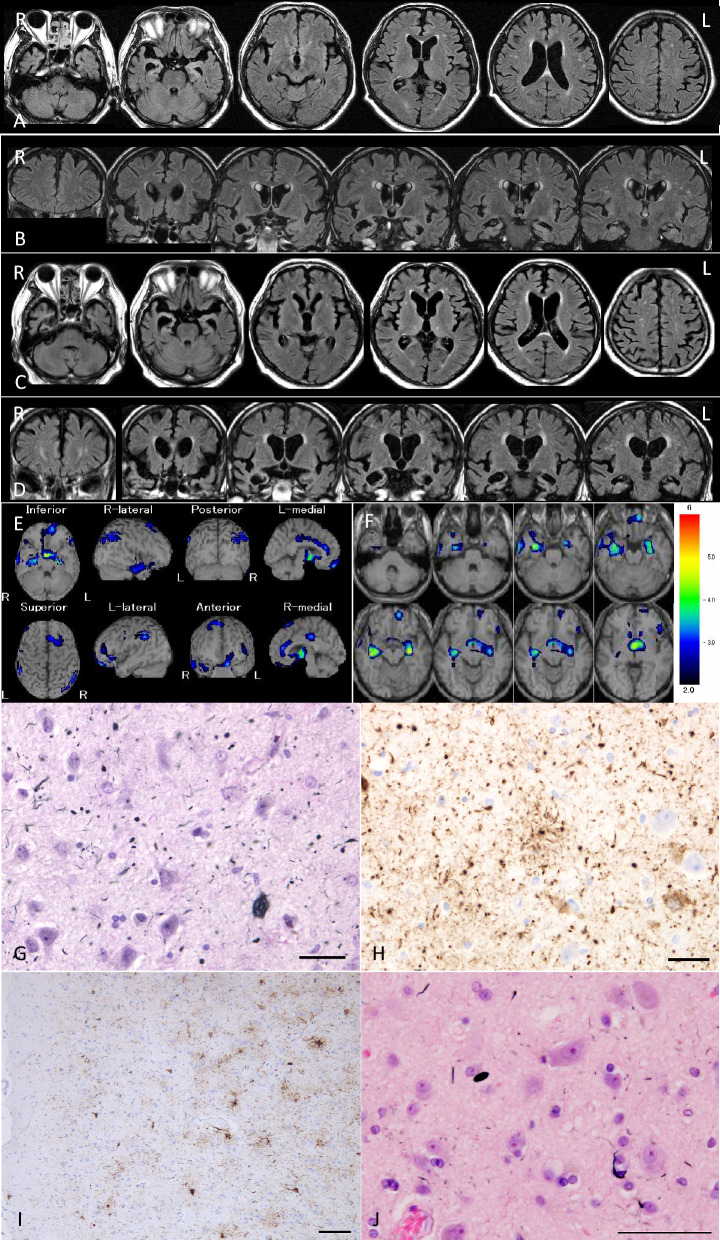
Radiological and pathological findings in case 2. A, B Axial (A) and coronal (B) MR images at the age of 69 years. The amygdala and adjacent parahippocampal gyrus show right side predominant atrophy. Temporal lobes are also atrophic, but frontal, parietal, and occipital cortices appear to be not involved. C, D Axial (C) and coronal (D) MR images at age 74. Right side-predominant atrophy in the amygdala and hippocampus become more evident. Mild frontal atrophy was also noted. E, F
